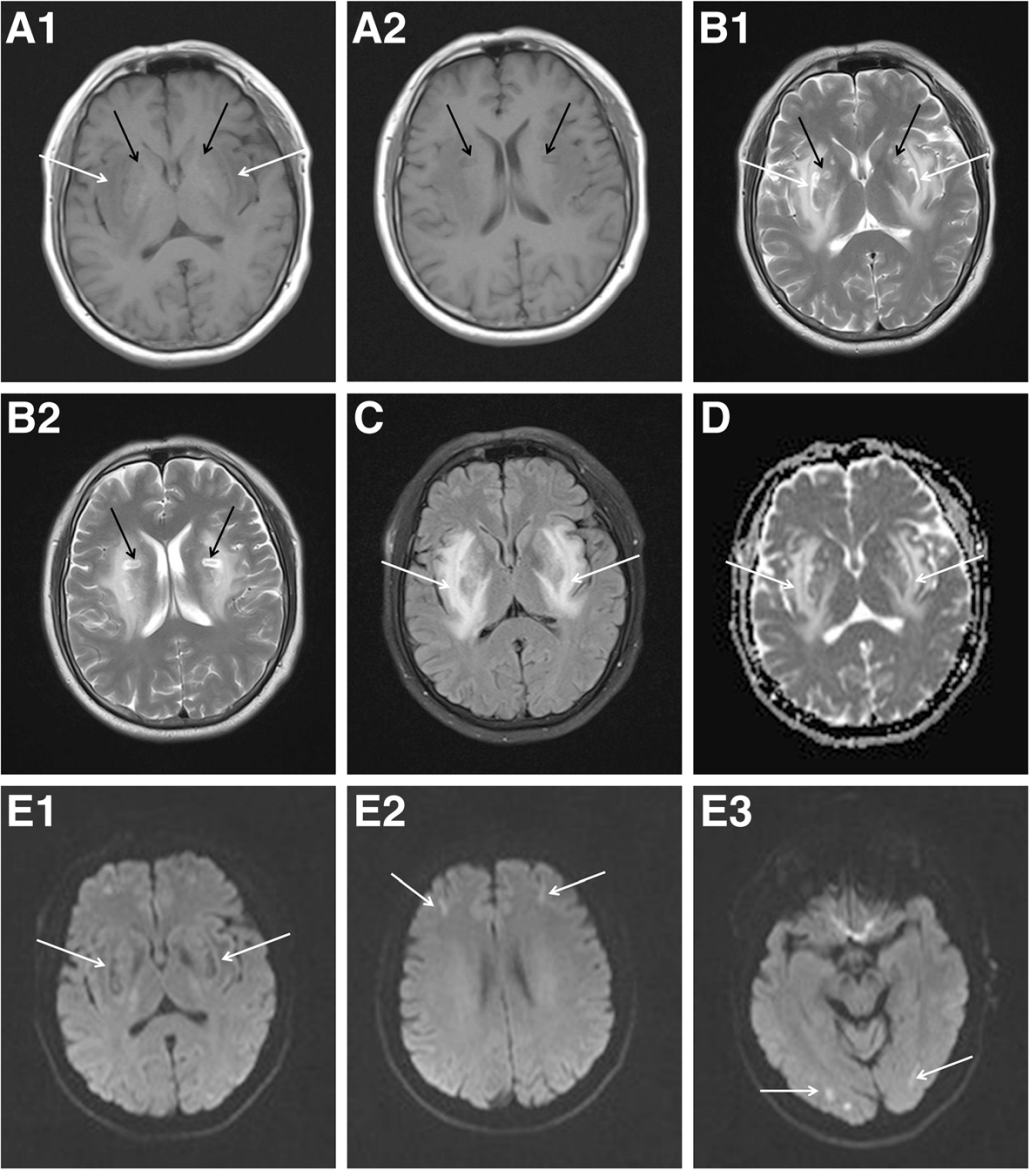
a1–e3. Follow-up magnetic resonance imaging obtained 25 days after admission showed symmetrical abnormal signals in the bilateral posterior limb of the internal capsule, putamen, extern al capsule, and insular lobe. The signals were hypointense on T1-weighted imaging sequence (T1WI) (a1, white arrows) and hyperintense on T2-weighted imaging sequence (T2WI) (b1, white arrows), (c) fluid-attenuated inversion recovery, and (d) apparent diffusion coefficient . They were not limited by diffusion on (e1) diffusion-weighted imaging (DWI). Strip and dot-like signals, which were isointense and slightly hypointense on T1WI (a1–2, black arrows) and hypointense on T2WI (b1–2, black arrows), can be observed in the lesions. (e2–3) DWI revealed bilateral hyperintensity in the frontal and occipital lobes
